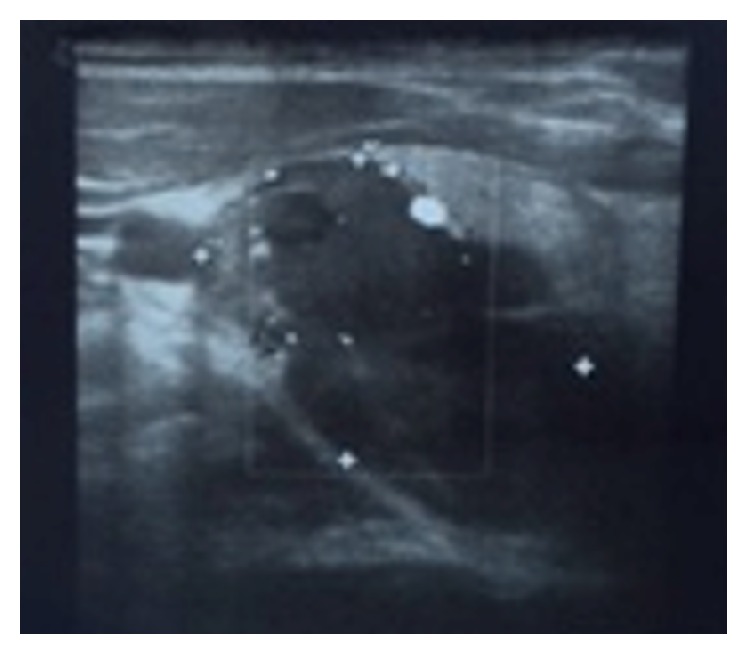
Thyroid ultrasound, large dominant complex 3.6 × 2.6 × 3.3 cm thyroid nodule with regular borders and peripheral vascularity in the right lobe.
